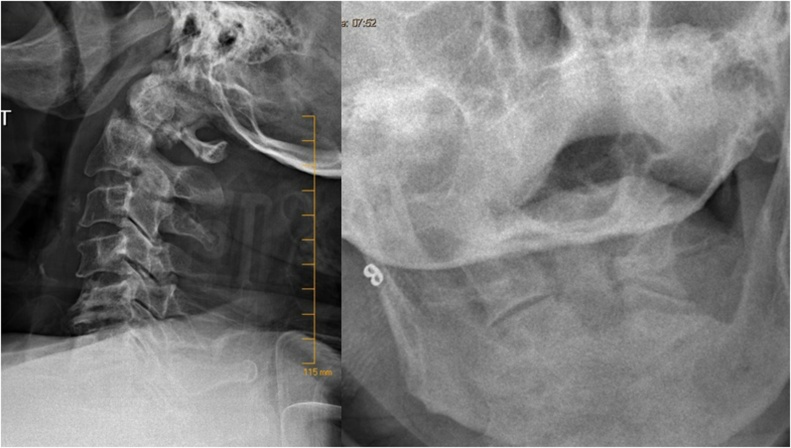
X-rays of the cervical spine (lateral and open mouth views) showing displaced and posteriorly angulated odontoid fracture.
